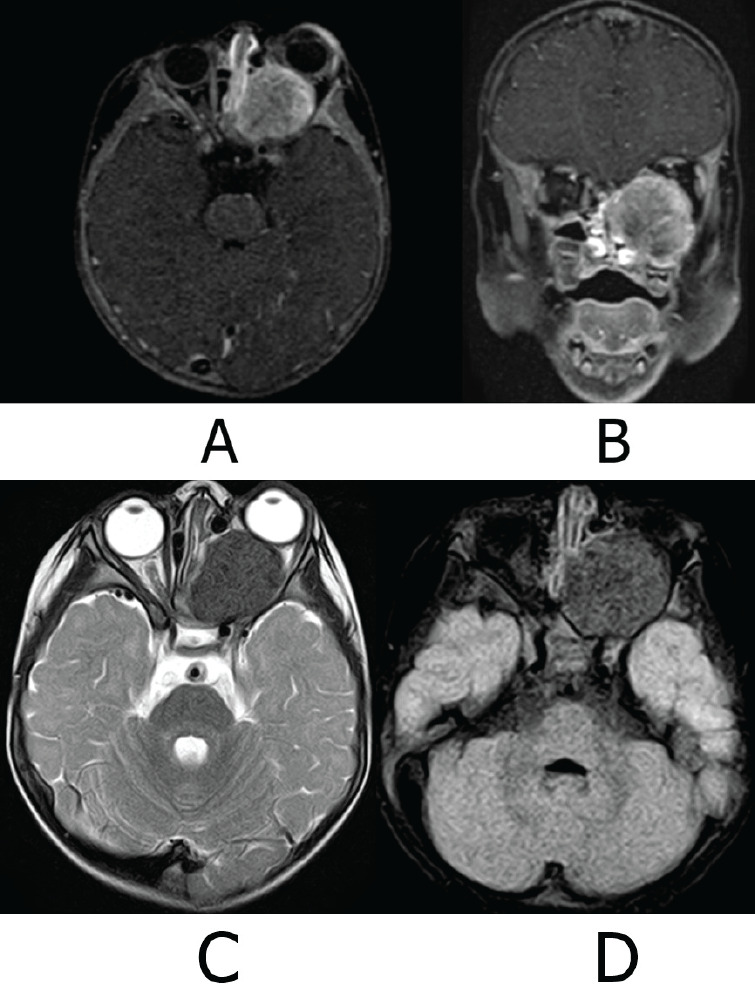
MRI of the skull. (a): Axial slice T1 Fs Gado sequence. (b): Coronal slice T1Fs Gado sequence. (c): Axial slice sequence T2. (d): Axial slice FLAIR Fs sequence. Ovoid tumour is with heterogeneous signal intensity in the different sequences due to the presence of calcified areas and heterogeneous reinforcement areas in post-gadolinear sequences. It conditions proptosis of the left eye, compressing and elongating the left optic nerve. There is no intracranial involvement, the cerebral parenchyma with adequate volume and gray matter-white matter differentiation, and there are no intra-or extra-axial lesions. Stem, gray nuclei and cerebellum without lesions, subarachnoid space and ventricular system normal. Source: Medical registry.
